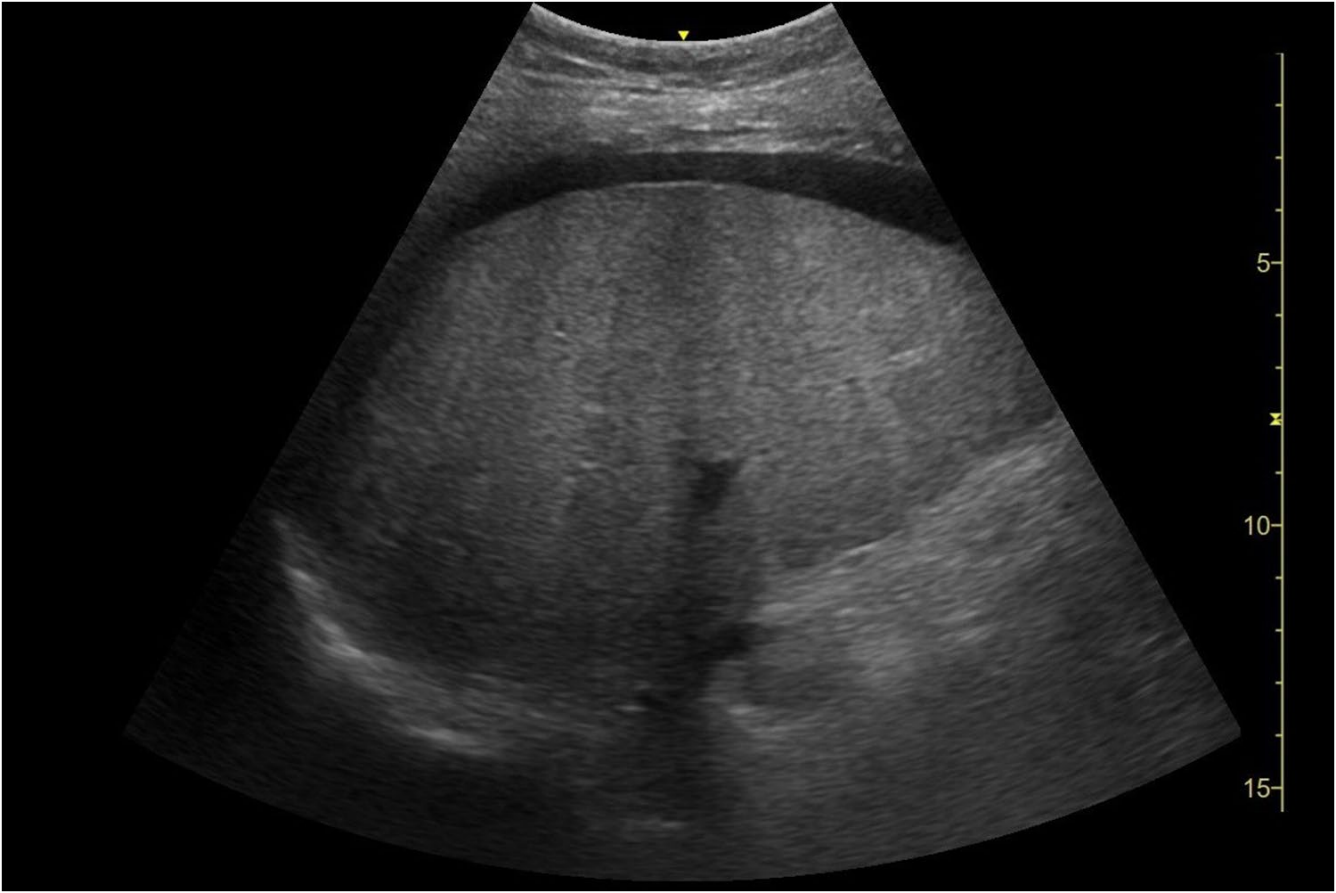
A sonographic image of the liver prior to biopsy. Minimal perihepatic ascites is present
